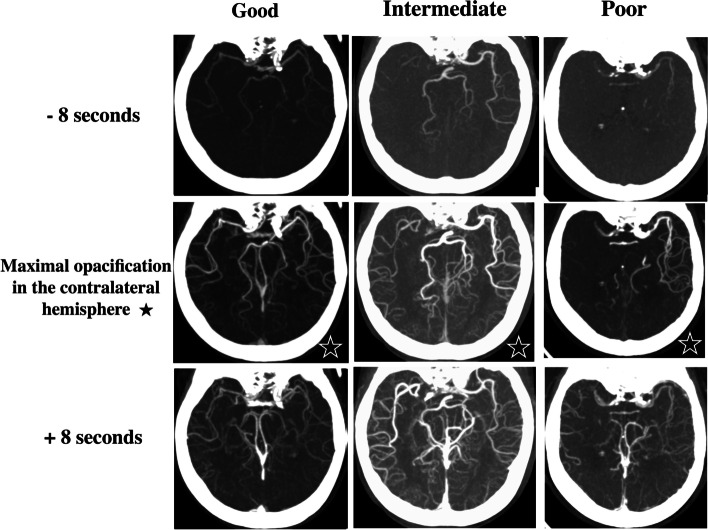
Collateral status based on dynax–CTA. Column: The collateral status was good, intermediate, and poor in the right M2 (left), right M1 (middle), and right M1 occlusion (right), respectively. Row: Time-resolved images showing 8 s (four frames) before maximal opacification of the MCA in the contralateral hemisphere (upper), maximal opacification of the MCA in the contralateral hemisphere (asterisk) (middle), and 8 s (four frames) after maximal opacification of the MCA in the contralateral hemisphere (lower). dynax–CTA, dynamic axial computed tomography angiography; MCA, middle cerebral angiography
